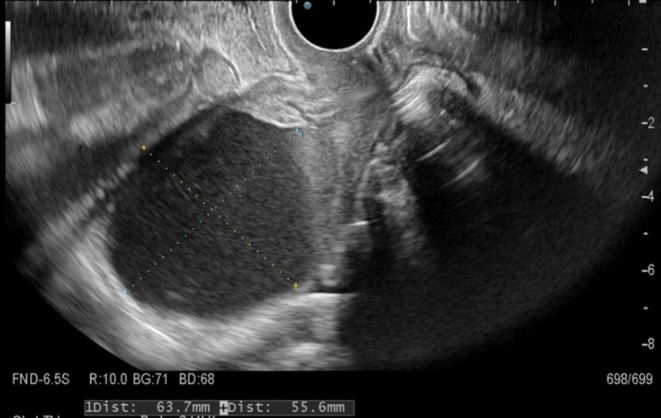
Transvaginal ultrasonography before ethanol sclerotherapy. Uterine cavity dilatation measuring 63 × 56 mm with a hypoechoic fluid collection and diffuse myometrial thinning is shown.
